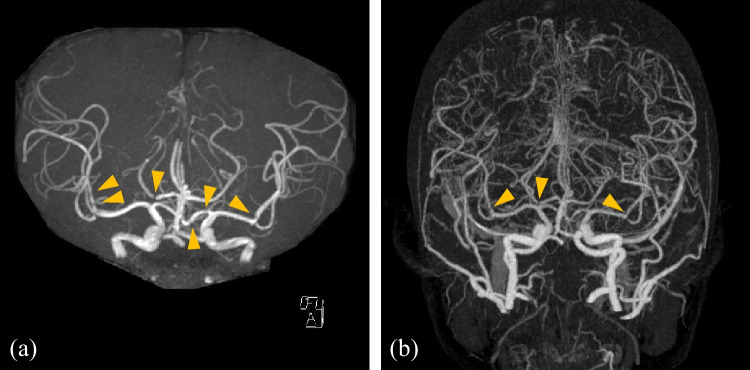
Findings of cerebral arteries. (a) Brain magnetic resonance angiography (MRA) findings. Brain MRA performed during hospital visit showed multiple and beaded-segmental vasoconstrictions in the cerebral arteries (arrow heads). (b) Brain contrast-enhanced computer tomography findings. Brain contrast-enhanced computer tomography performed one week after MRI showed multiple and beaded-segmental vasoconstrictions in the cerebral arteries (arrow heads)
